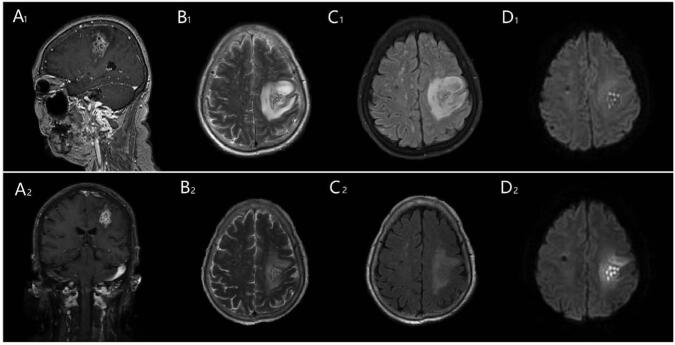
Longer signal on T1-weighted images on frontal parietal region of sagittal position and longer signal on T2-weighted images in the left frontoparietal junction area. (A1 and B1). Hyperintense in FLAIR and DWI sequence (C1 and D1). Multiple high signals with cluster distribution can be seen in the lesion area, with surrounding vasogenic edema and local mass effect. The same lesion area was reduced and edema was alleviated (A_2_–D_2_).
